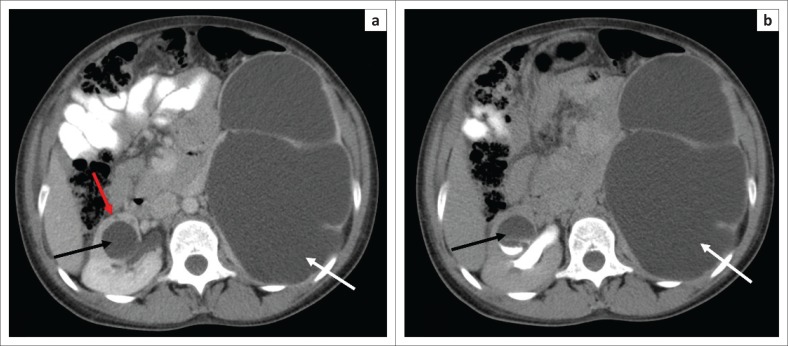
(a) Venous phase computed tomography (CT) abdomen demonstrates severe left hydronephrosis (white arrow) because of stricture in the pelvi-ureteric junction, with complete effacement of the renal parenchyma. A parenchymal cavity (black arrow) is present in the right kidney with surrounding heterogeneous low attenuation (red arrow) suggestive of pyelonephritis. (b) Delayed phase CT abdomen demonstrates contrast filling of the right renal cavity suggestive of communication with the calyceal system. The left kidney is non-functional.
